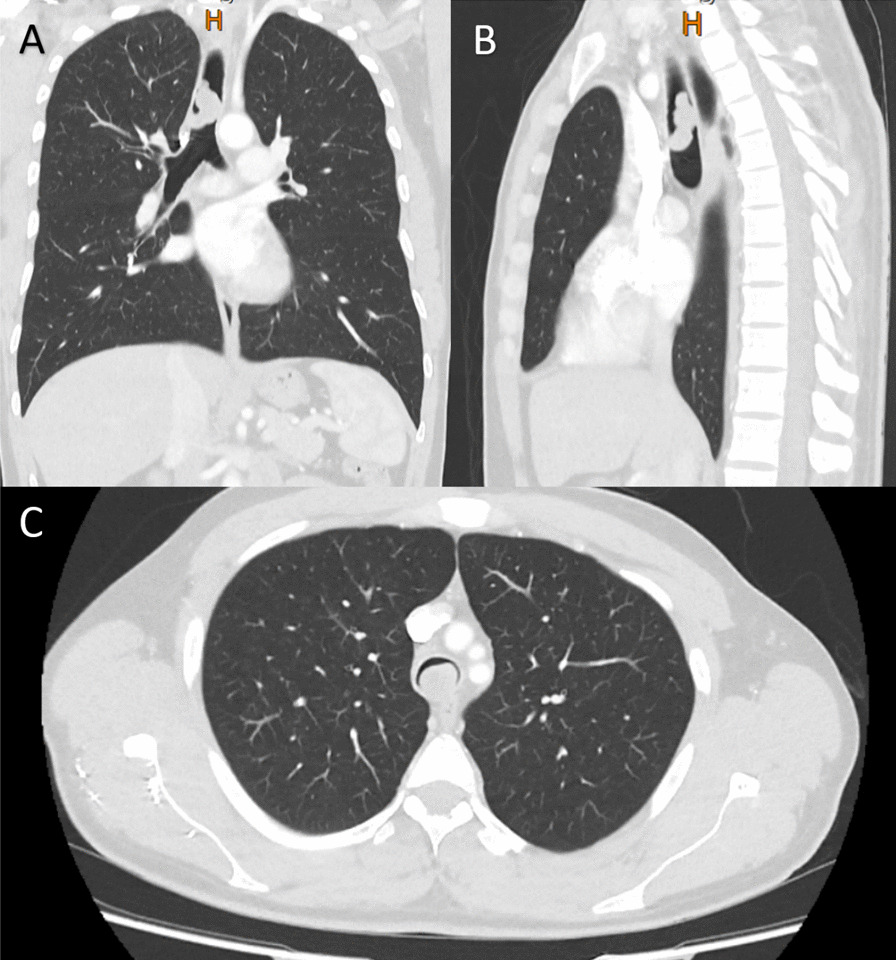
The computed tomography scan of a 38-year-old male with an endotracheal mass and near-totally obstructing the tracheal airway in its distal part; A coronal view, B sagittal view, and C axial view
